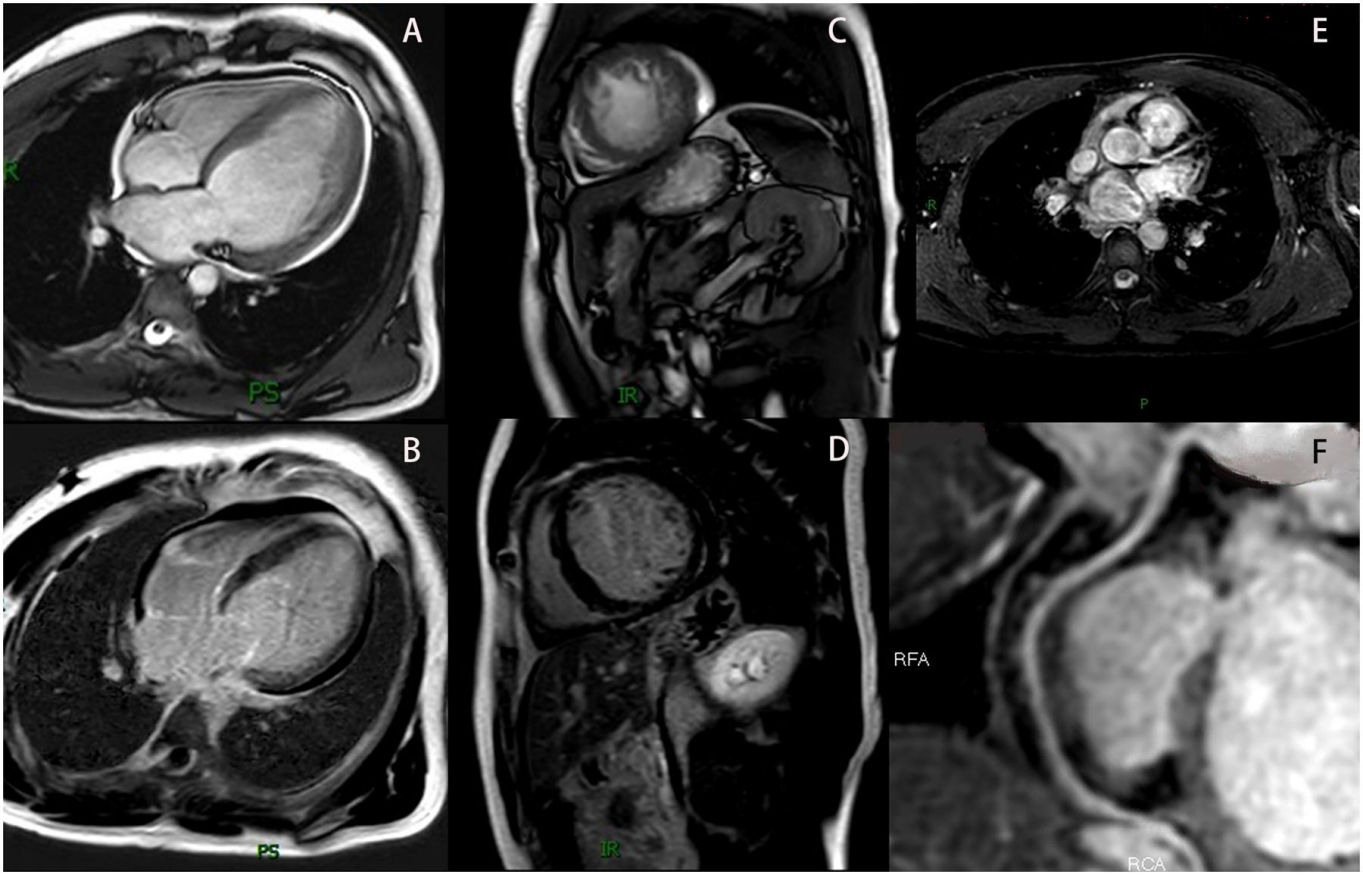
The patient's MRI results. (A) Show the cardiac MRI four-chamber image. (B) Show the LGE image in the four-chamber view. (C) Show the cardiac MRI short-axis image. (D) Show the LGE image in the short-axis view. (E) Show the LAD in the CMRA (The LCX was not visualized due to its diminutive caliber). (F) Show the RCA in the CMRA. MRI, magnetic resonance imaging; LGE, late gadolinium enhancement; LAD, left anterior descending artery; LCX, left circumflex artery; RCA, right coronary artery coronary; CMRA, coronary magnetic resonance angiography.
